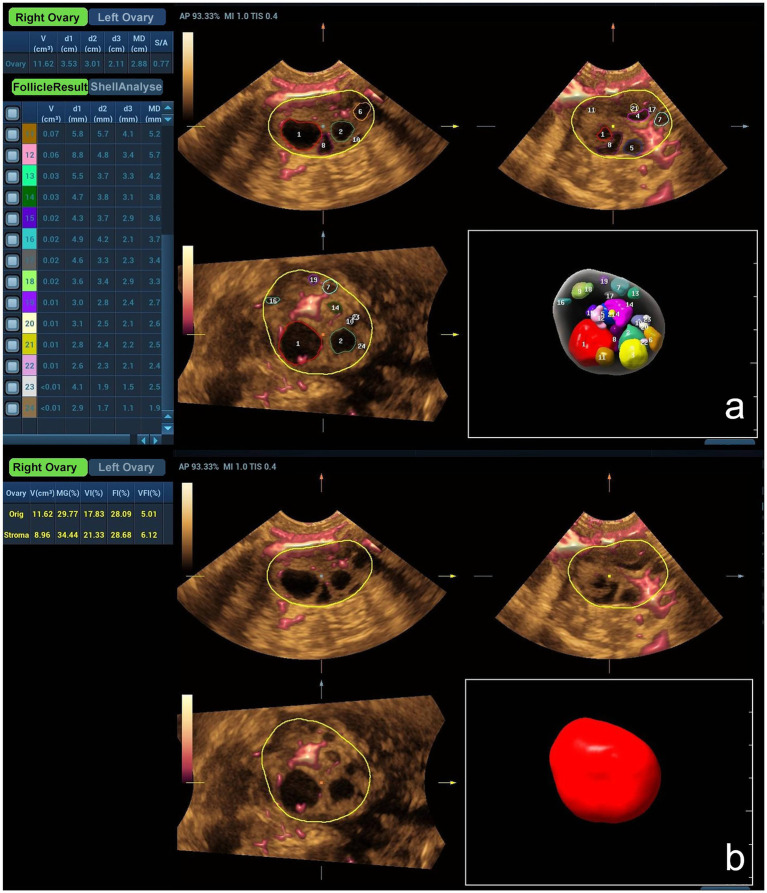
(a)Transvaginal three-dimensional automatic follicle measurement and ultra-microvascular flow imaging of right ovary follicles in a normal patient. (a) Automatic follicle measurement shows multiple antral follicles (numbered 1–24) in the right ovary (yellow outline), and the left panel lists each follicle’s indicators (OV, diameter d1/d2/d3, mean diameter MD, etc.). Color Doppler imaging shows the vascular distribution around the follicles. (b) Shows the ultra-microvascular flow and three-dimensional volume imaging (red structure) of the same right ovary (yellow outline), and the left panel lists origin and stromal OV, MG, VI, FI, and VFI.
